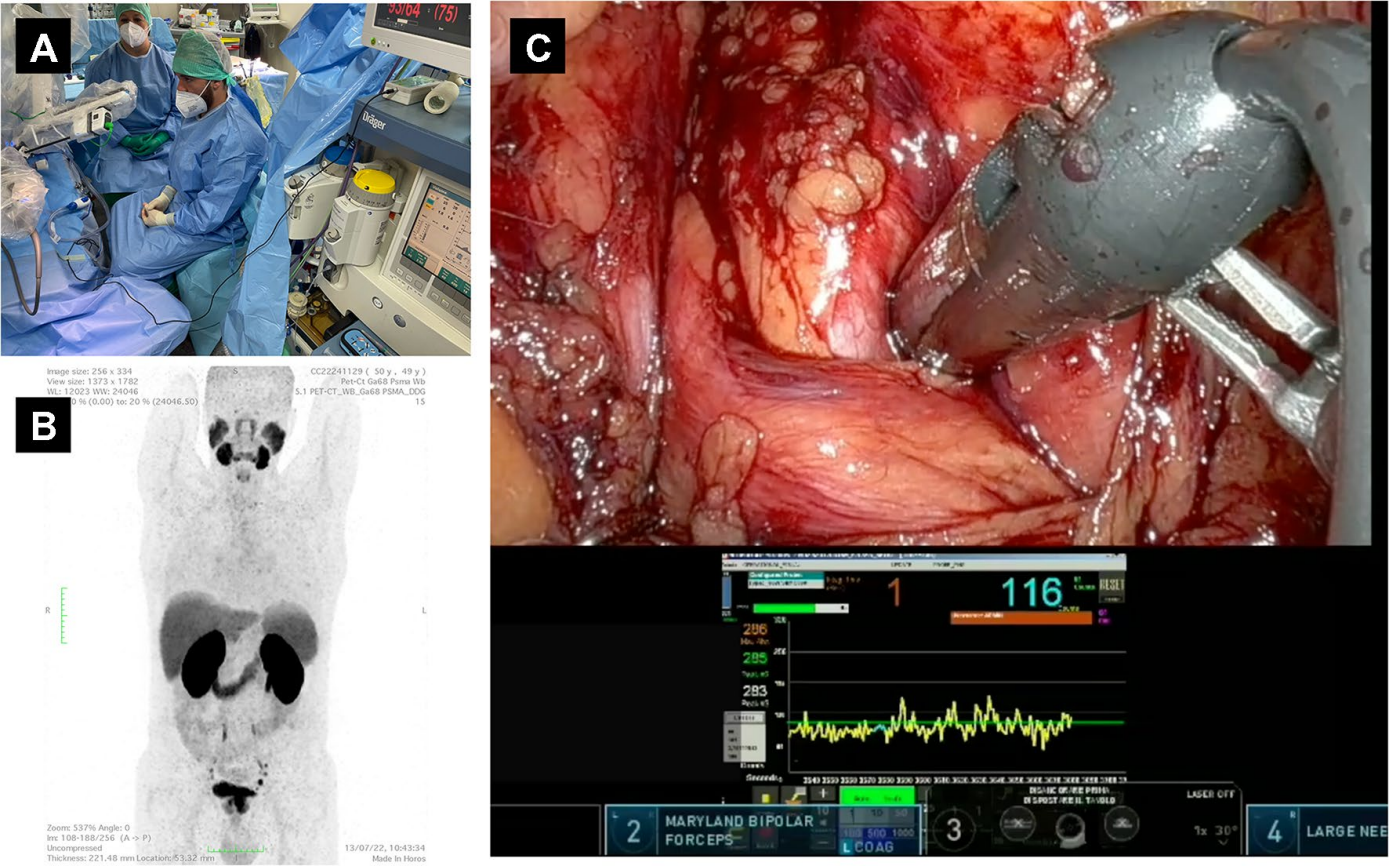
A Operating room during one of the procedures: the black cable coming from the drop-in probe can be distinguished. B MIP image of ^68^ Ga-PSMA-PET of one of the considered patients (Pt. #3). C View of the da Vinci monitor as seen by the surgeon during the procedure (Pt. #5), including the TilePro split-screen option to view the probe measurements directly in the surgical console
